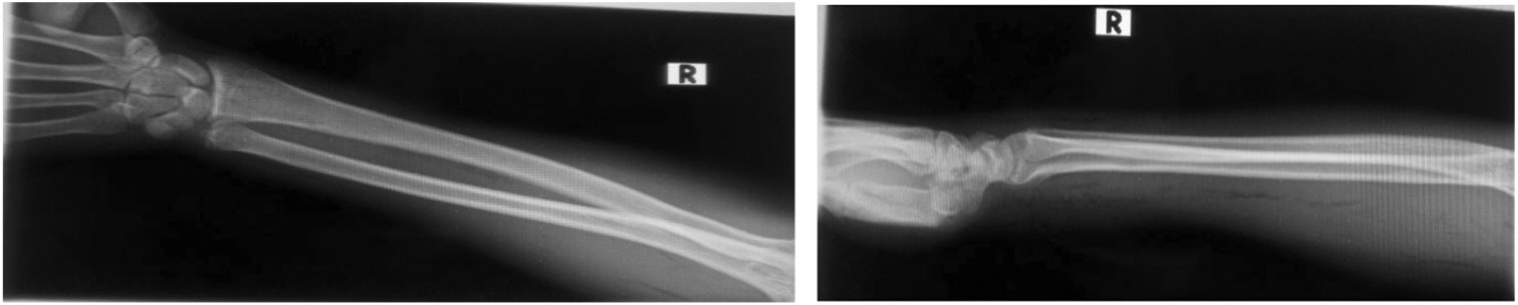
Radiographs demonstrating subcutaneous gas in the forearm.
